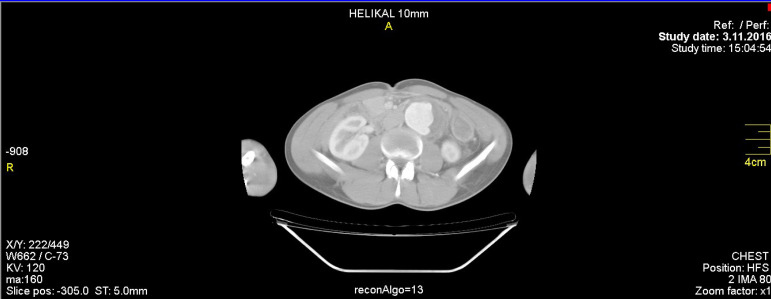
Preoperative thoracoabdominal computed tomography. Thoracoabdominal aortic aneurysm.
